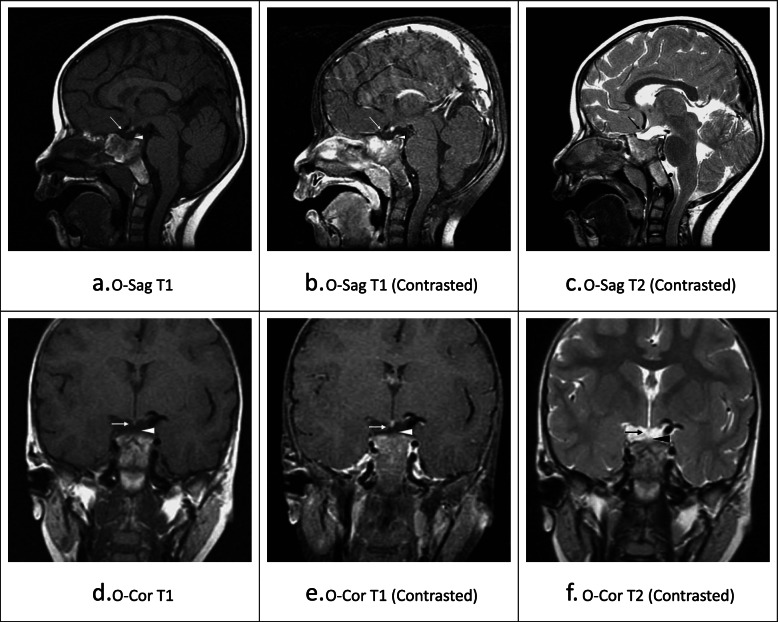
The sella magnetic resonance imaging (MRI) of the patient. The MRI (a-f) showed atrophic anterior pituitary lobe (arrow), disruption of pituitary stalk and ectopic posterior pituitary lobe (arrowhead) at the junction of the pituitary stalk and hypothalamus. No evidence of abnormal signal intensity mass lesion in the sella and suprasellar region was noted. The MRI scan series was labeled below each image. O-Sag = orthogonal sagittal; O-Cor = orthogonal coronal
